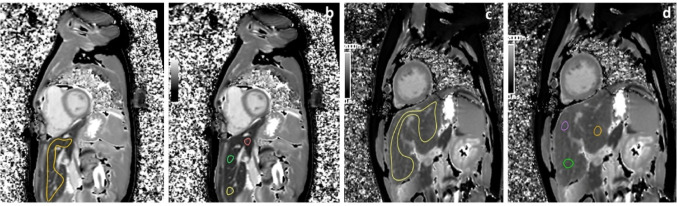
T1 maps of patients with repaired TOF (a, b) and with Fontan circulation (c, d). Sagittal MRI slices demonstrating two measurement approaches: a single wide ROI positioned over the largest homogeneous region of the hepatic parenchyma (a, c); and multiple small circular ROIs distributed across different homogeneous areas of the hepatic parenchyma (b, d). *TOF* tetralogy of Fallot, *MRI* magnetic resonance imaging, *ROI* region of interest
